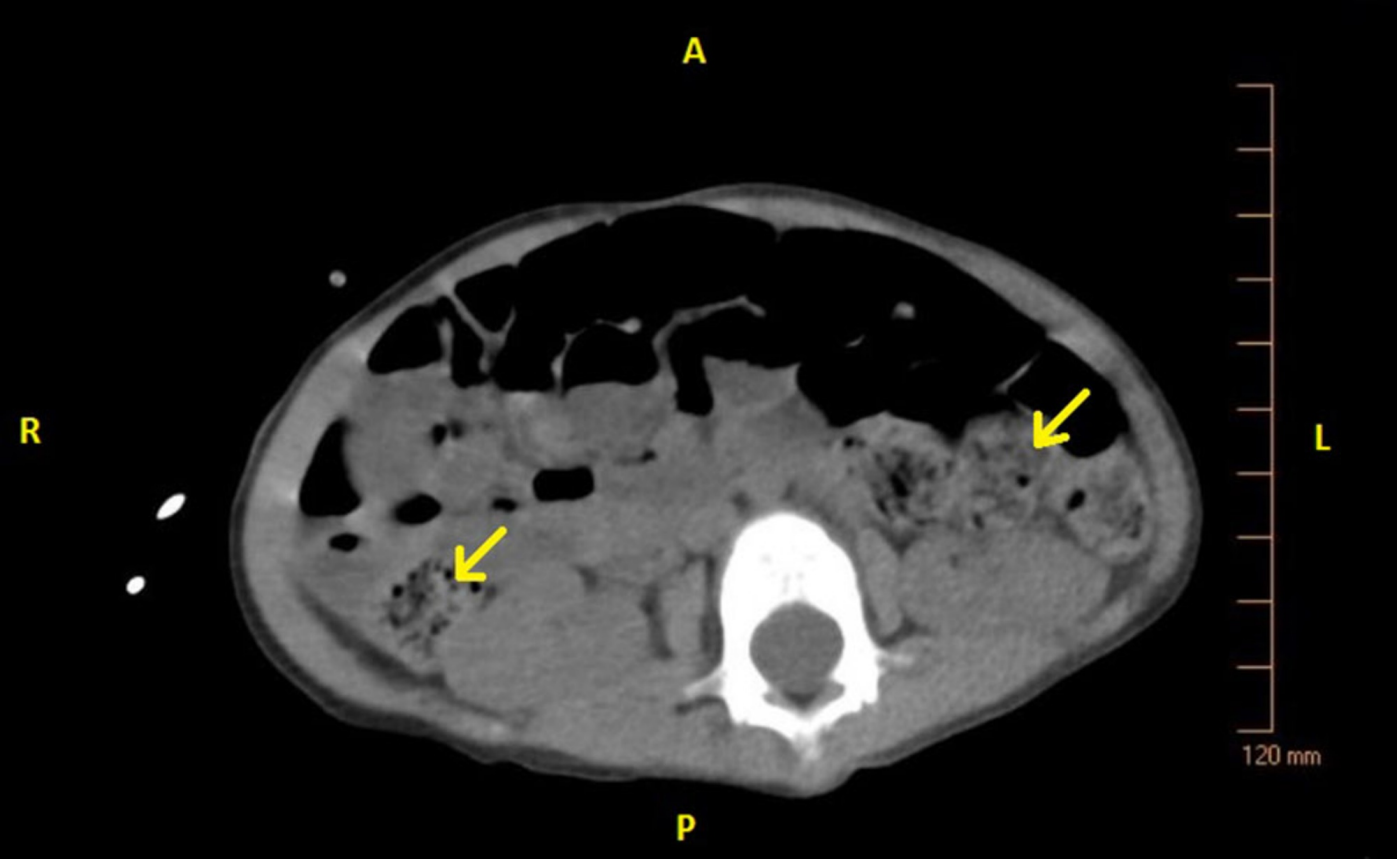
CT abdomen non-contrast, transverse view: large amount of stool and gas throughout the colon (arrows). A, Anterior; P, Posterior; L, Left; R, Right; CT, computed tomography
